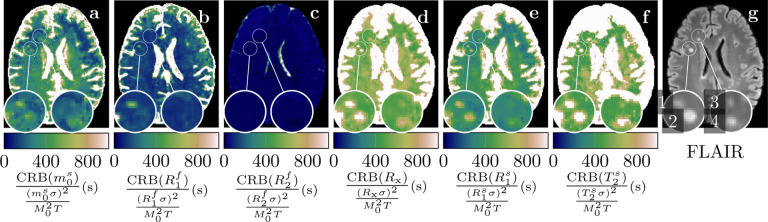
Cramér-Rao bound (CRB) of the in vivo parameter estimates shown in Fig. 6a–f, normalized to resemble the inverse squared SNR of the parameter estimates (cf. Tab. 1). For the CRB calculations, we used the unconstrained MT model and the corresponding estimates. The FLAIR image is provided for anatomical reference.
